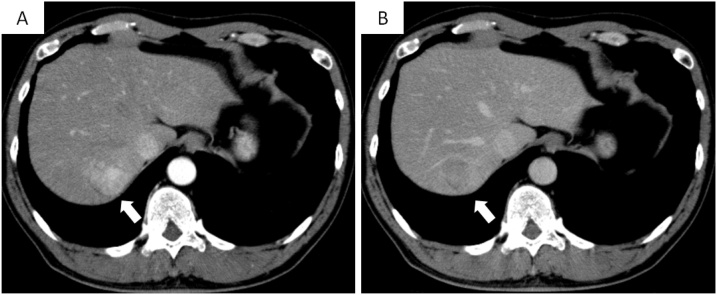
Tumor is enhanced in the arterial phase (A), followed by a washout in the late phase (B) on contrast-enhanced computed tomography (arrow).
